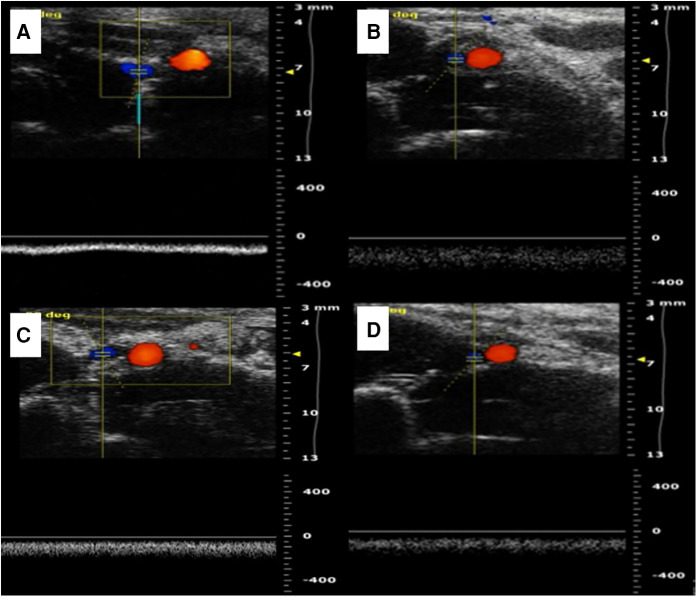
Two kinds of vascular ultrasound in vein replacement model. (A) Abdominal ultrasound at 1 week after operation in the cuff group. (B) Abdominal ultrasound at 4 weeks after operation in the cuff group. (C) Abdominal ultrasound at 1 week after operation in the suture group. (D) Abdominal ultrasound at 4 weeks after operation in the suture group.
